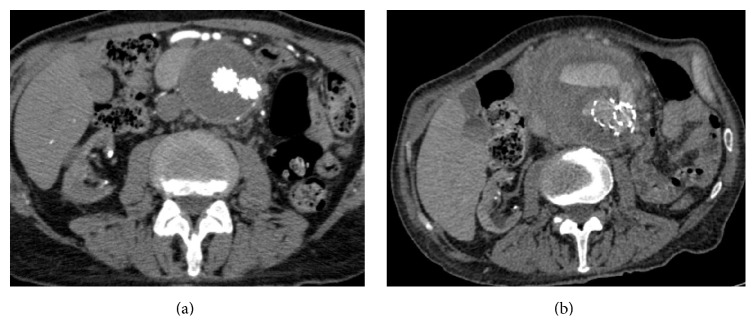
(a) 12-month follow-up CT scan indicating complete exclusion of the aneurysm with sac shrinkage after the initial procedure. (b) CT angiography showing enlarged AAA with hematoma and endoleak originating from distal main body of the EVAR graft.
